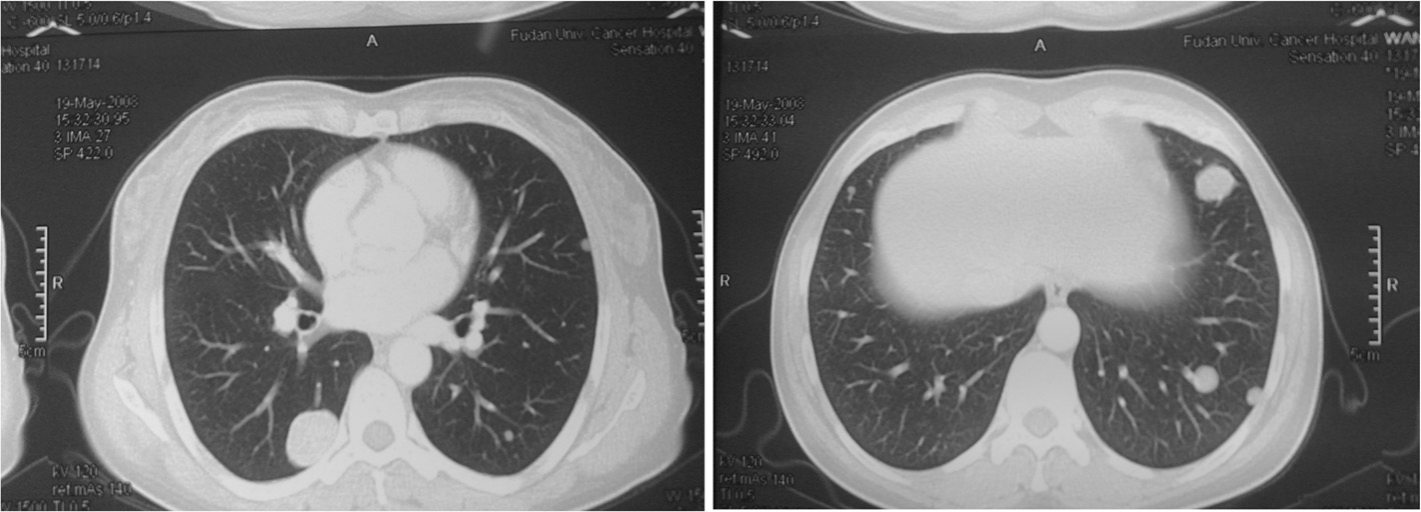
Computed tomography scan shows that lesions are generally smooth and diffusely distributed in both lungs.
